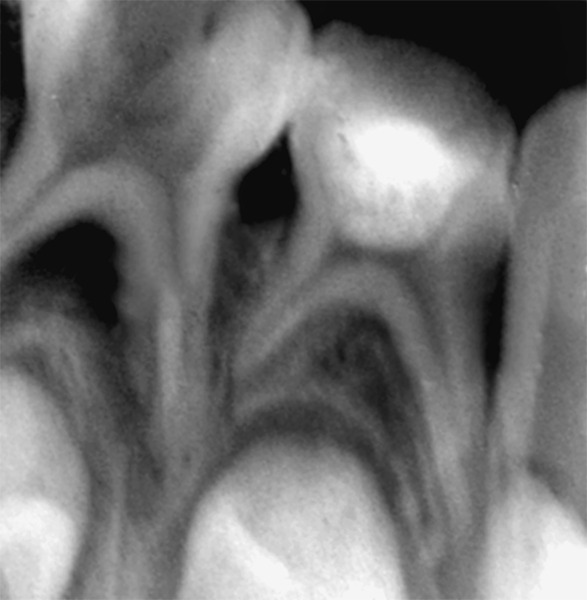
Postoperative radiograph in relation to 74
